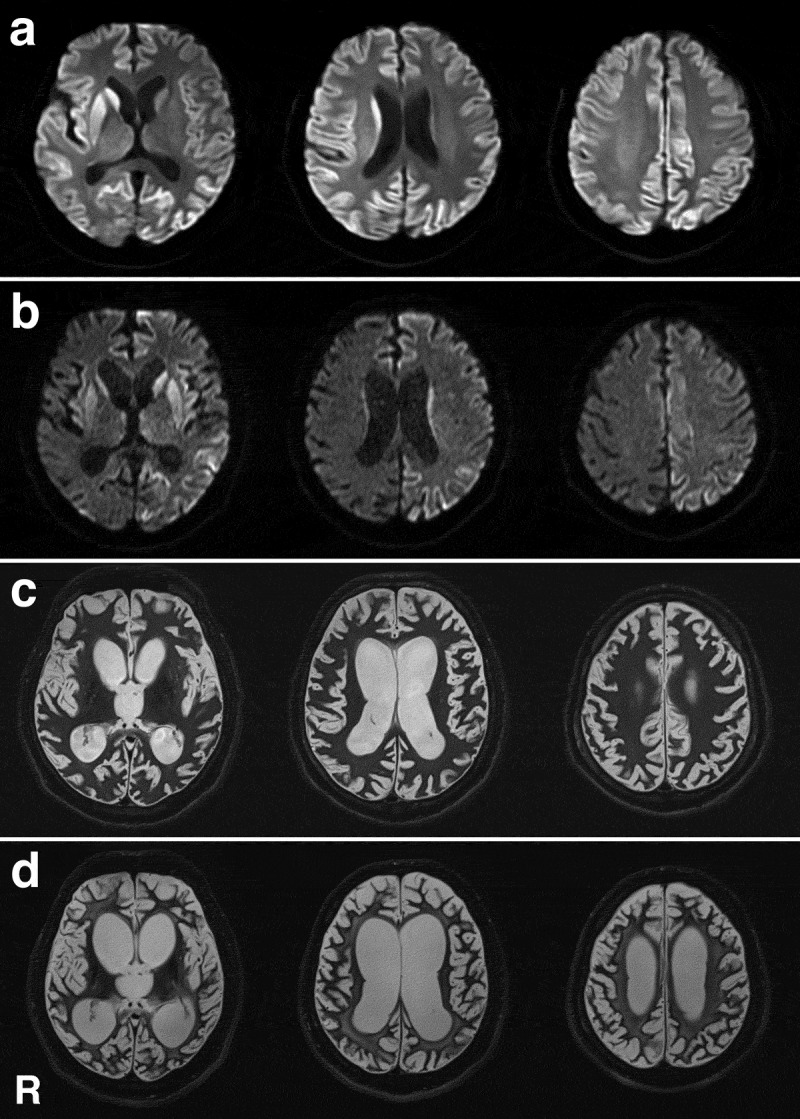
Magnetic resonance images of the brain. (a) Diffusion-weighted images (DWI) obtained 2 weeks after symptom onset show extensive hyperintense regions in the cerebral cortical ribbon and striatum, particularly on the right side. (b) DWI obtained 4 months after symptom onset shows extensive hyperintense regions of the cerebral cortex and striatum, particularly on the left side. Brain atrophy is mildly progressed. (c) T2-weighted images obtained 12 months after symptom onset show general cerebral atrophy and lateral ventricular dilatation. White matter degeneration is still not apparent. (d) T2-weighted images obtained 24 months after symptom onset show severe cerebral atrophy and lateral ventricular dilatation. White matter degeneration is recognized. R, right side
